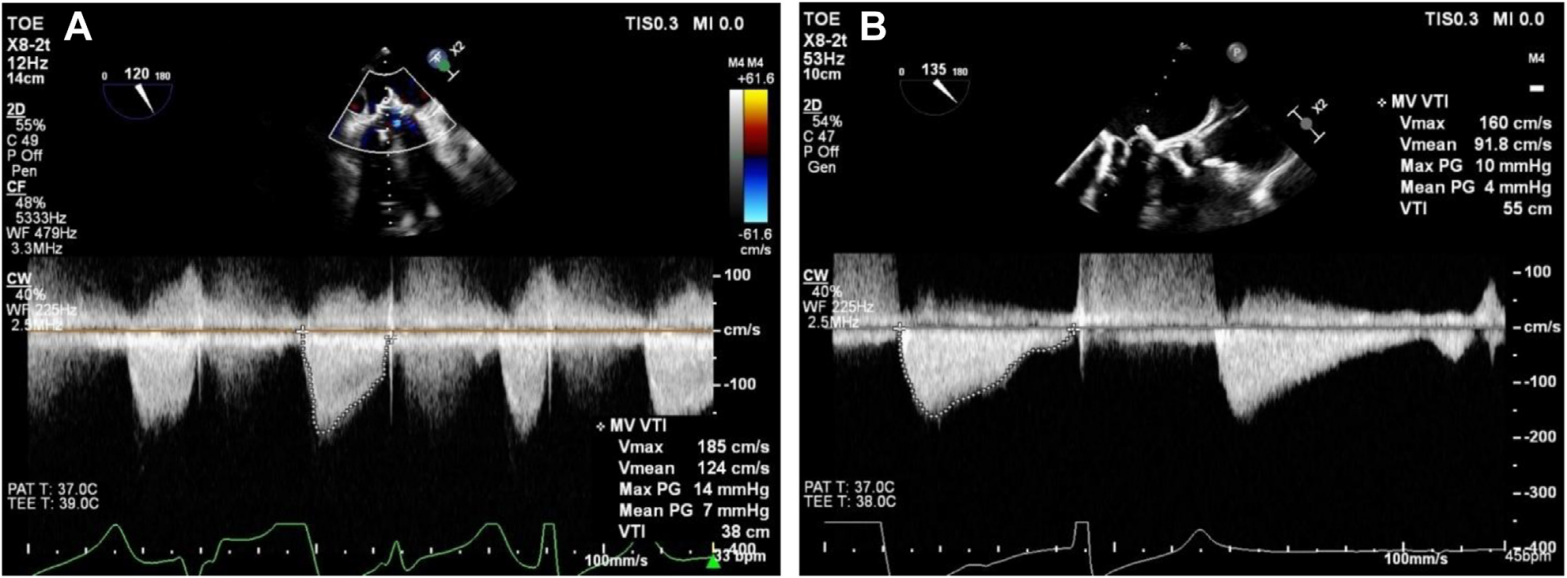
Two-dimensional TEE, midesophageal view with color flow Doppler–guided continuous-wave spectral Doppler display, demonstrates (A) high MV mean pressure gradient (7 mm Hg, heart rate 33 beats/min) before mitral ViViV insertion and (B) reduced MV mean pressure gradient (4 mm Hg, heart rate 45 beats/min) immediately following mitral ViViV implantation.
